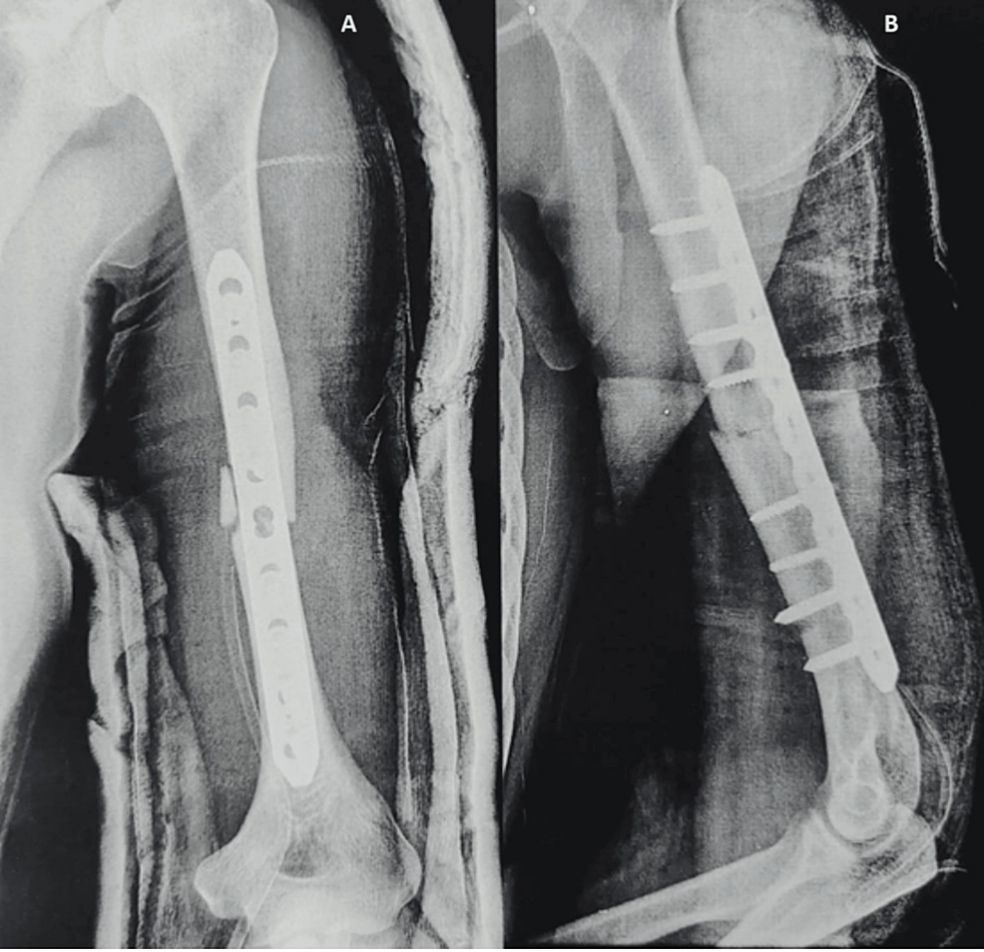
Postoperative radiographs of humerus shaft fracture fixed with dynamic compression plating (A) Lateral view; (B) anteroposterior view
